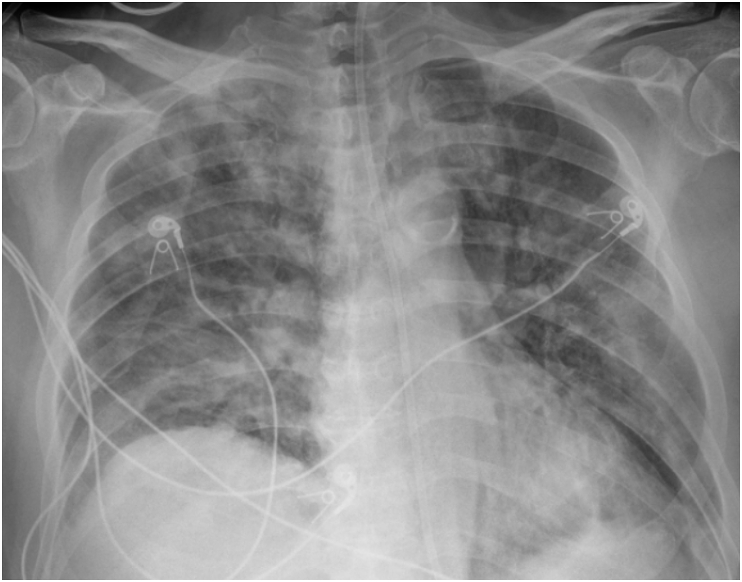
Chest radiograph of Case 2 on hospital day 4 with slight improvement in multifocal airspace opacities.
